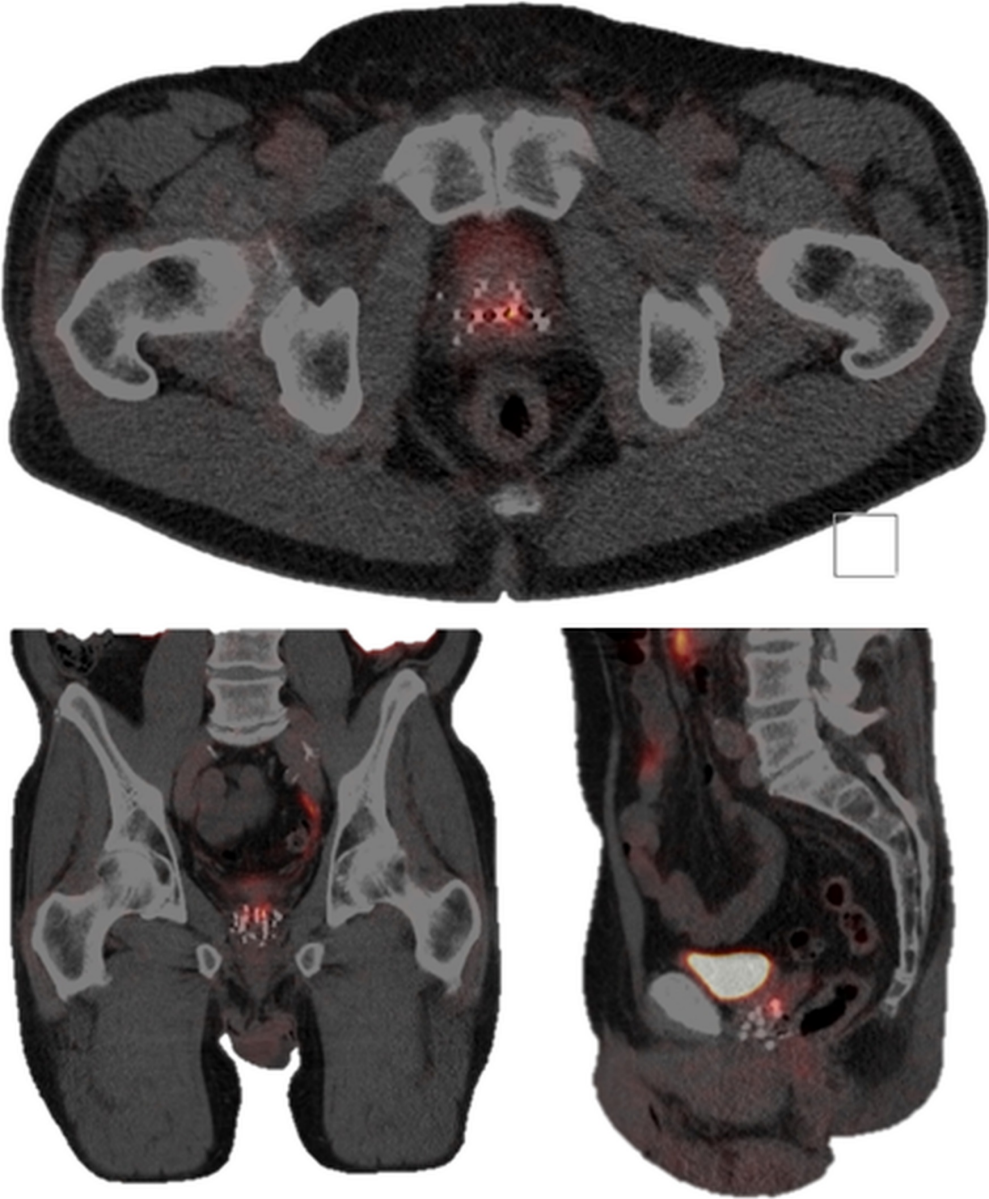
Second recurrence lesion on 68Ga-PSMA PET-CT Transversal plane (upper image), coronal plane (lower-left image), and sagittal plane (lower-right image), showing the second recurrence lesion in the left dorsal peripheral zone on the 68Ga-PSMA PET-CT, 11 years after initial prostate cancer treatment. PSMA PET-CT: prostate-specific membrane antigen positron emission tomography-computed tomography
